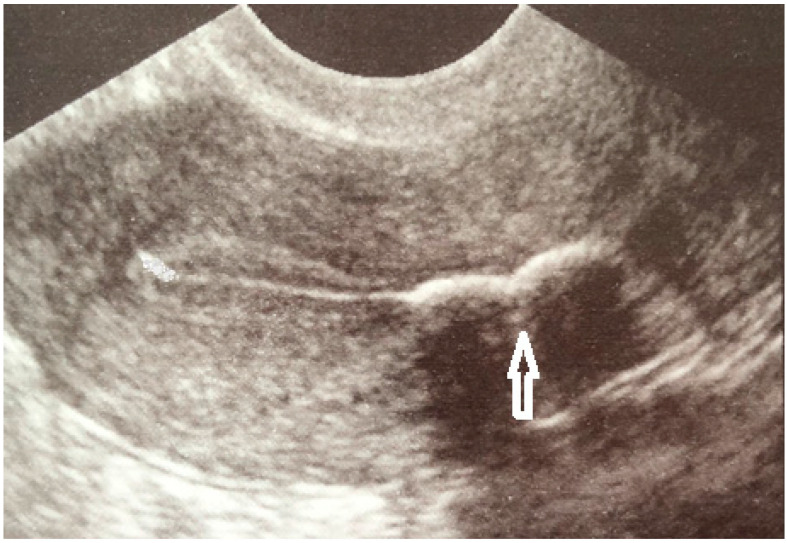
Ultrasound image of intrauterine calcification.
